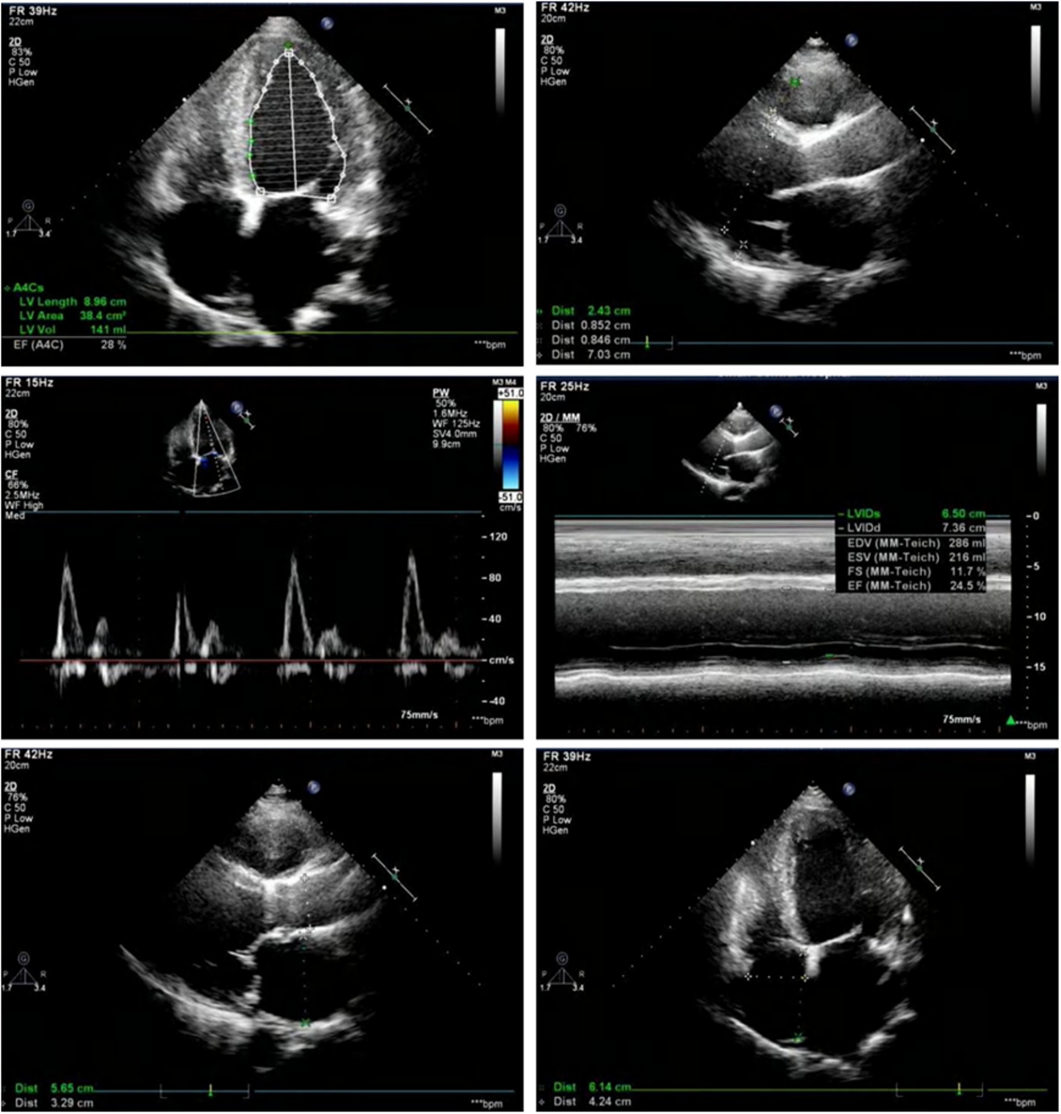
Echocardiography examination of HF cases.
